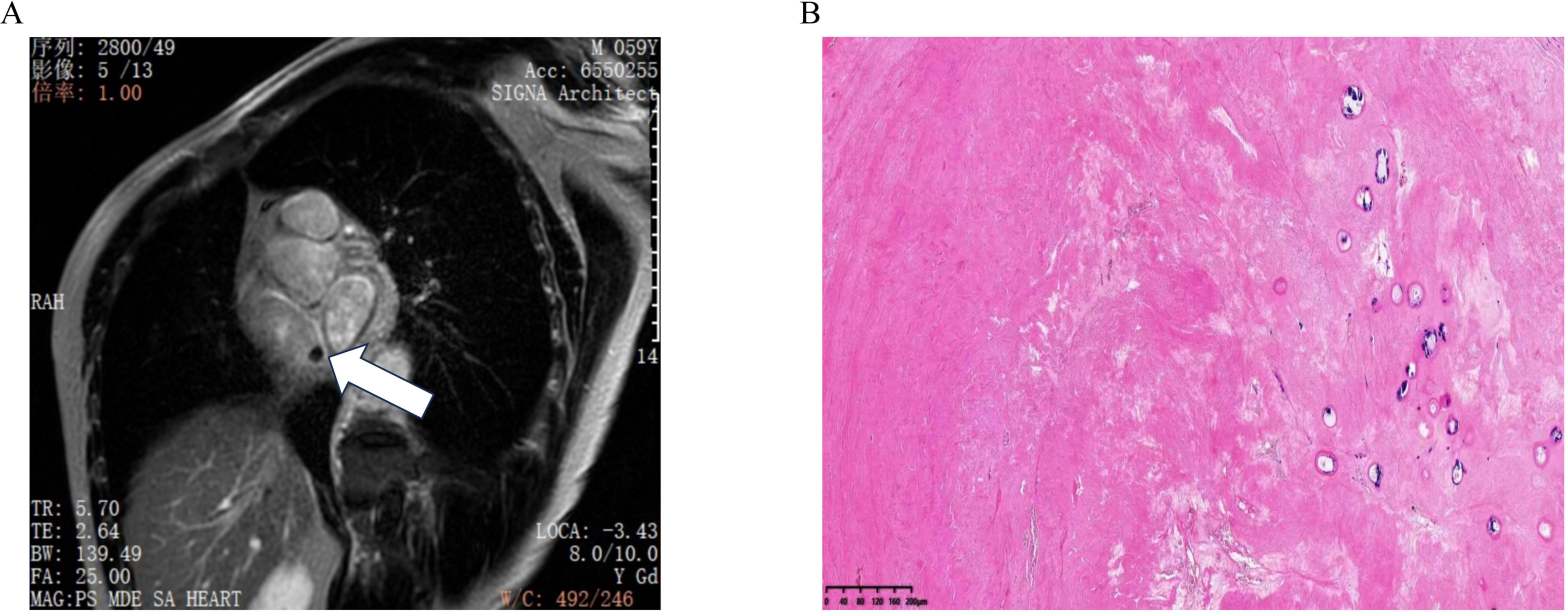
(A) Cardiac MRI shows a nodule at the top of the right atrium near the opening of the inferior vena cava, with no enhancement observed after contrast administration. (B) Pathology result shows fibrous tissue proliferation and collagenization, consistent with thrombus tissue.
